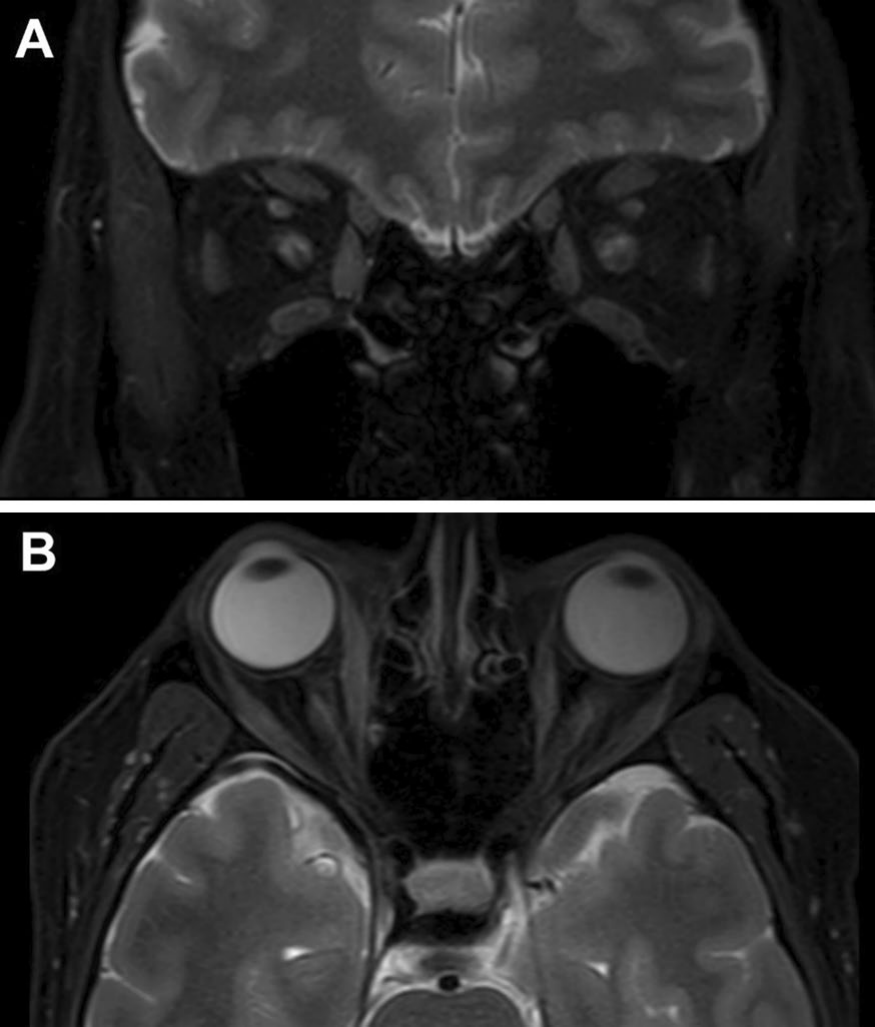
T2-weighted, fat-suppressed MRI scans for Case 2. A (coronal) and B (axial) demonstrate the predominant asymmetric thickening of the right medial rectus with tendon involvement evidenced by T2 hyperintensity, along with bilateral enlargement of the lateral and inferior recti
